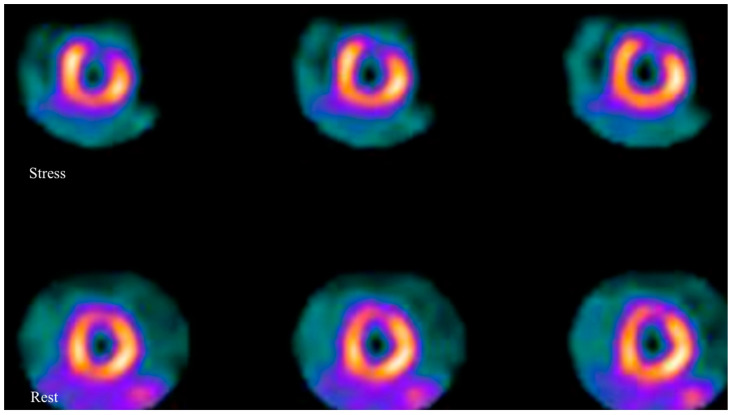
Axial images of a myocardial scintigraphy performed in a pediatric patient with KD: images show a severe reversible perfusion defect in the anterior wall of left ventricle, which is suggestive for myocardial ischemia.
